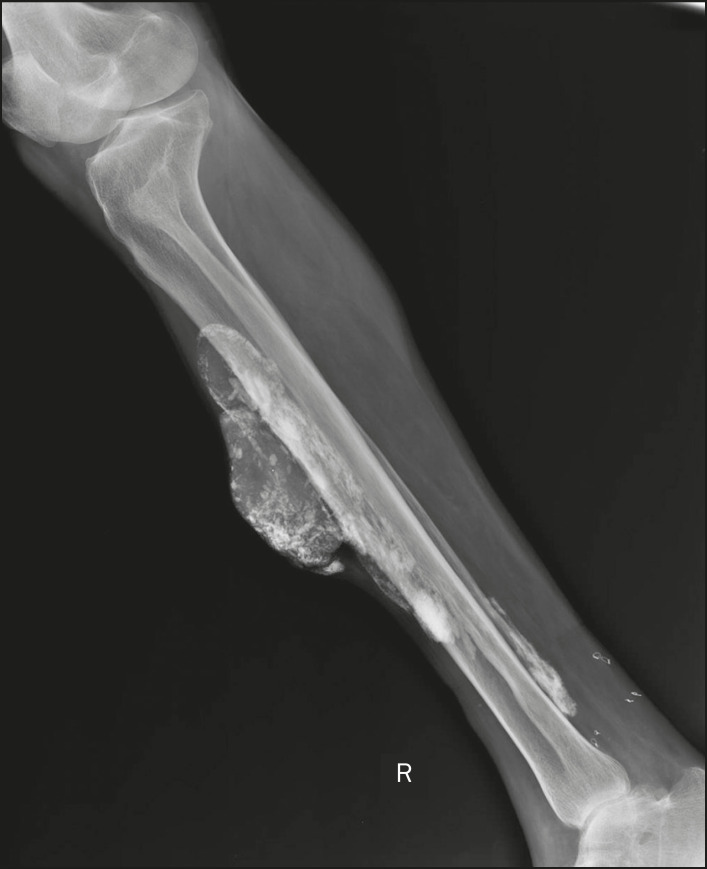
Muscle calcifications in calcified muscle hematoma. Lateral X-ray of the leg showing a mass with predominantly peripheral soft-tissue calcifications, corresponding to a muscle hematoma with dystrophic calcifications.
